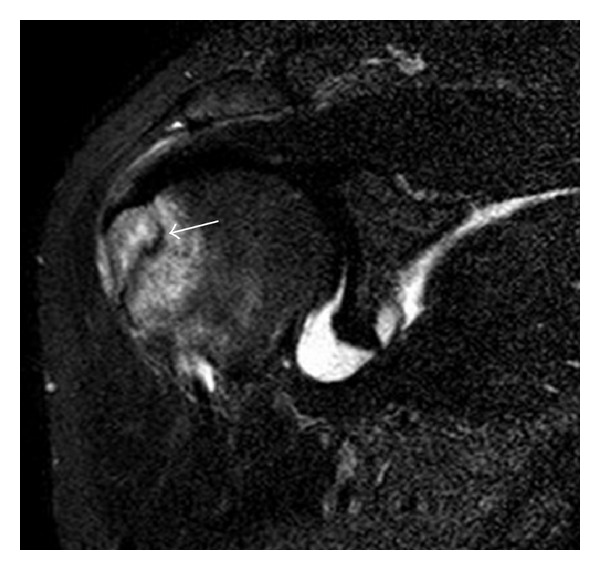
Traumatic fracture of the greater tuberosity in a 51-year-old man presenting with left shoulder pain after a fall on ice. Initial radiographs were normal. Coronal inversion recovery MRI shows a fracture line (arrow) through the greater tuberosity surrounded by a bone marrow edema pattern.
